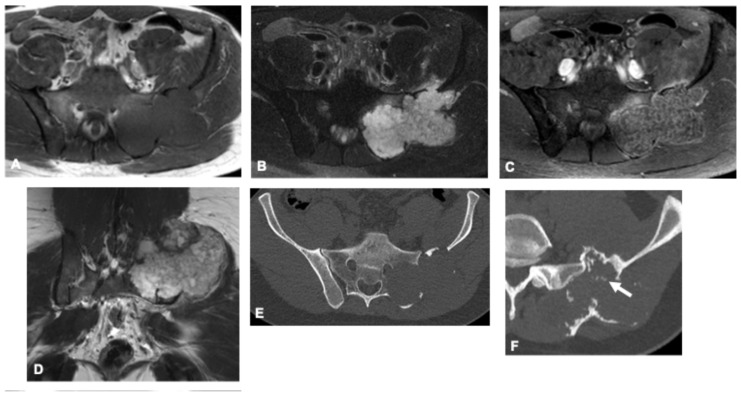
Chondrosarcoma in a 31-year-old man. Axial and coronal MR images (A–D) show an extensive osteolytic lesion involving the left sacral ala and iliac bone, demonstrating intermediate signal intensity on the T1-weighted image (A) and high signal on T2-weighted images (B,D) with thin hypointense internal septations and a characteristic lobulated contour. Post-contrast imaging reveals peripheral and septal enhancement (C), consistent with the cartilaginous nature of the tumor. Corresponding CT images (E,F) highlight the relative paucity of matrix mineralization, most pronounced in the cranial portion (arrows), typical of chondral-type matrix calcifications.
